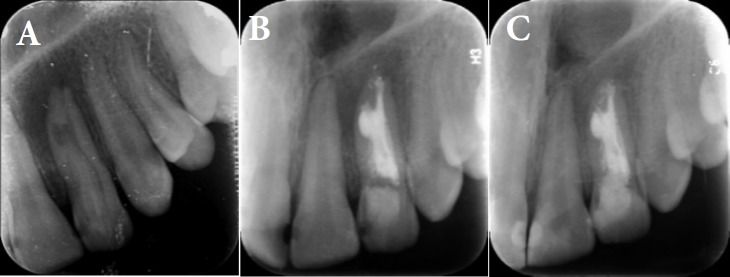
A)* Preoperative radiography of the maxillary left lateral incisor; *B) *Postoperative radiography immediately after treatment; *C)* Postoperative radiography after one year follow up
